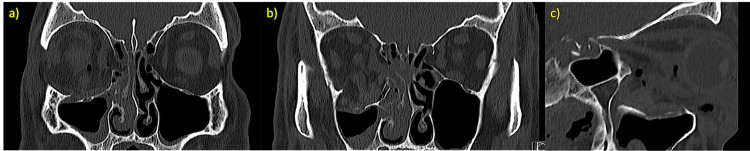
Initial CT scan demonstrating a right orbital bone fracture and herniation of orbital contents (a) Coronal view, anterior aspect. (b) Coronal view, posterior aspect. (c) Sagittal view.
